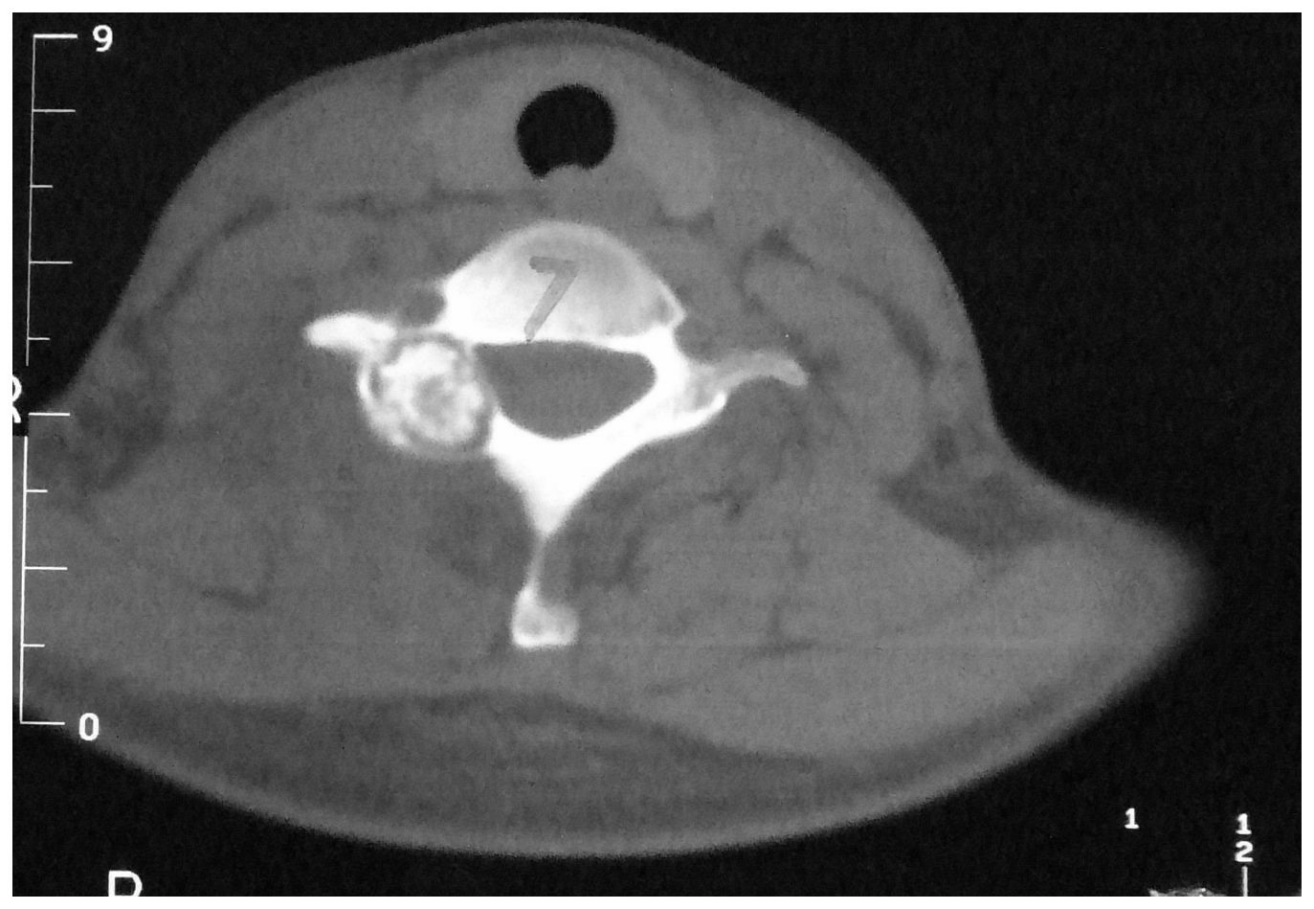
Preoperative CT scan shows a lesion located at the posterior elements of the C7 vertebra. There is an expansile lesion with cortical destruction and surrounding sclerosis. The boundaries are unclear and mottled densities of calcification can be seen within the lesion.
